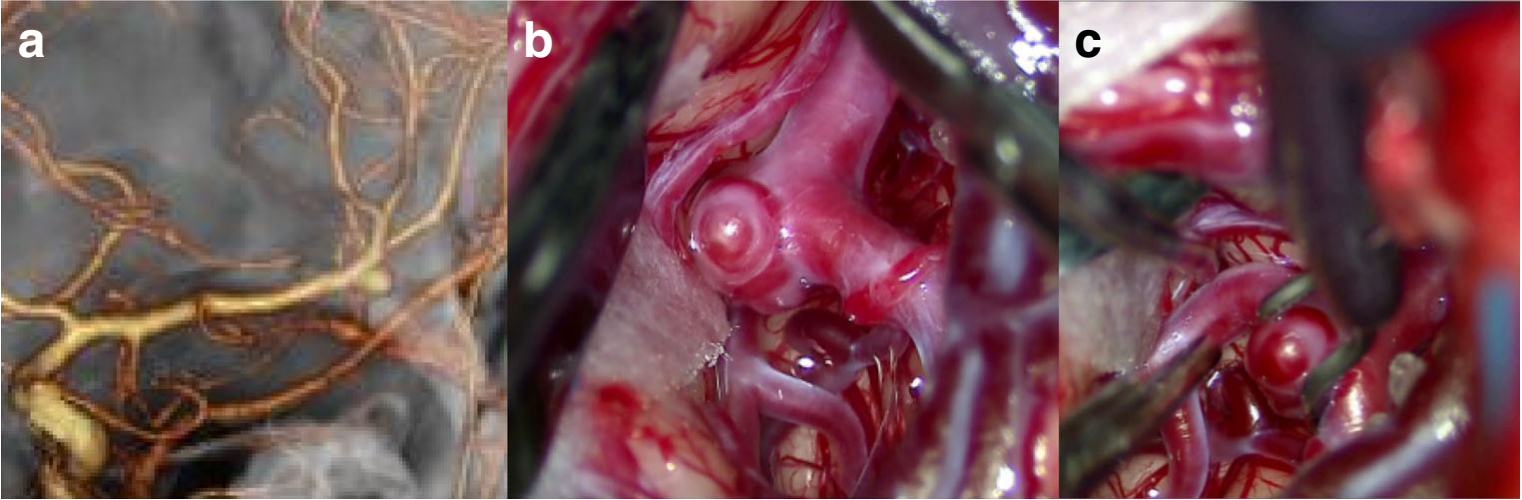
Representative case of a left MCA aneurysm. a Preoperative 3D–CT angiogram. b, c Intraoperative views
